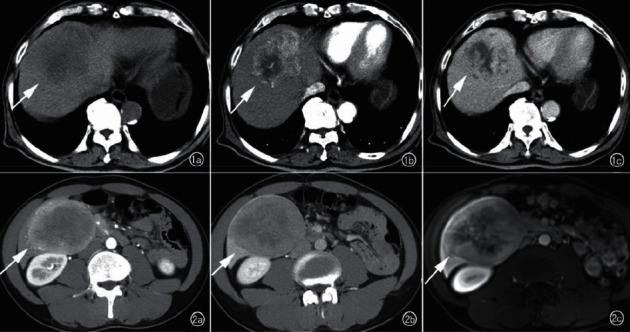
Axial MRI images of a patient with HCC in the left lobe of the liver. (a) A T2 mixed slightly high signal mass is seen in the left lobe of the liver; (b) significant inhomogeneous enhancement in the arterial phase; (c) contouring and separation in the portal vein phase (arrows).
